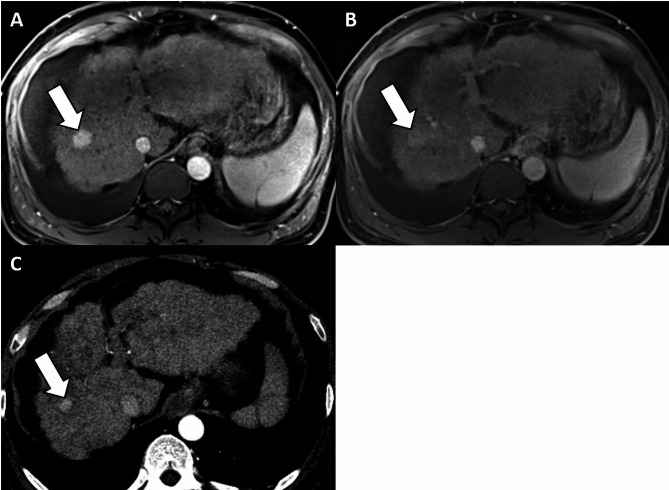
Images of typical threshold growth in a 47-year-old man with hepatocellular carcinoma. (a) Arterial phase hepatobiliary contrast agent-enhanced axial MRI scan shows an 18 mm observation with non-rim hyperenhancement in segment 8 of the liver (arrow). (b) Portal venous phase axial MR image shows no washout or enhancing capsule (arrow). Accordingly, this observation was categorized as LR-4. However, the same observation measured 11 mm on an arterial phase axial CT image obtained 4 months before (c), corresponding to threshold growth. Considering the presence of additional major features of threshold growth, the observation was recategorized as LR-5. Hepatocellular carcinoma was confirmed in the explanted liver. CT, computed tomography; MRI, magnetic resource imaging
